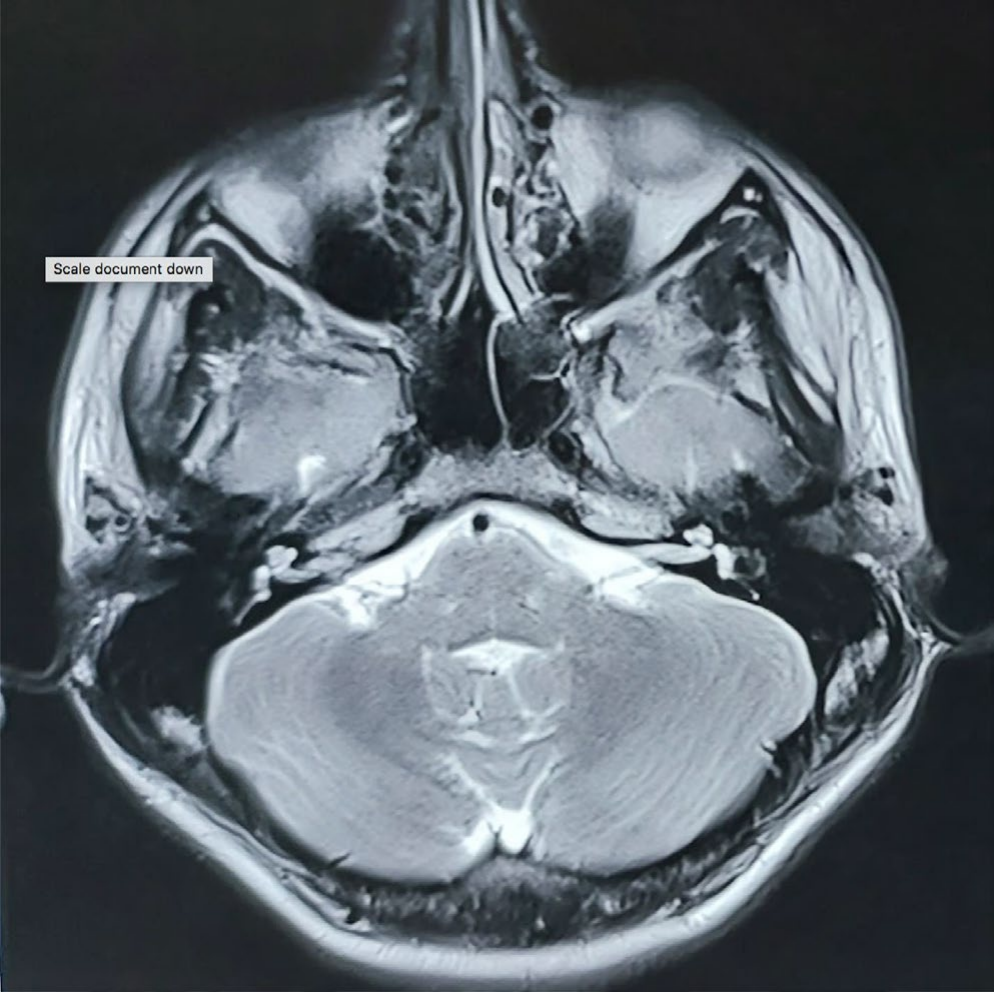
T2 MRI brain showing normal finding
